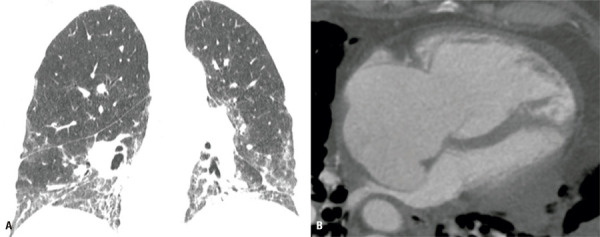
A 55-year-old man with rheumatoid arthritis. Axial chest computed tomography images show (A) ground glass and reticular opacities in a nonspecific interstitial pneumonia pattern, and (B) marked right chamber enlargement and leftward bowing of the interventricular septum due to pulmonary hypertension
